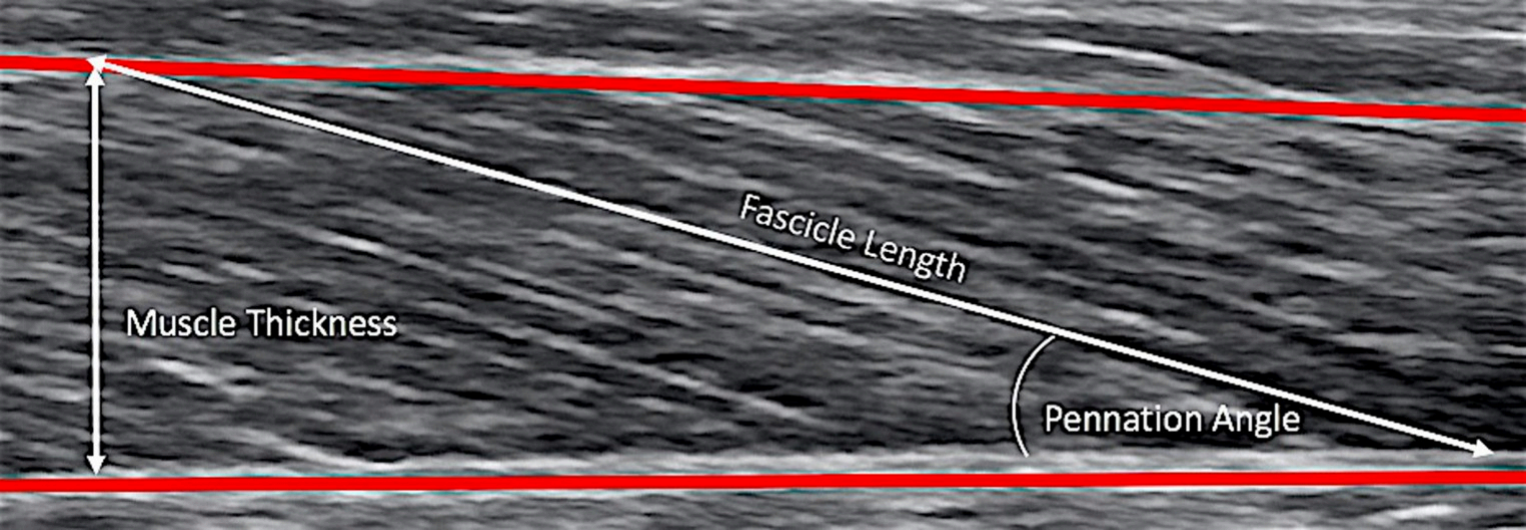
Ultrasonographic (longitudinal) image for measuring muscle thickness, pennation angle and fascicle length in vastus lateralis.
